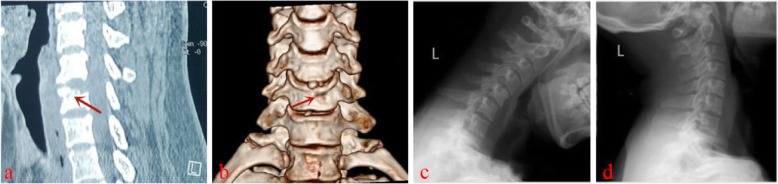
A 6-month postoperative CT examination revealed that the bone tunnel had healed and no fracture occurs (a, b). The last follow-up X-ray radiographs in flexion and extension positions of cervical spine(c, d) showed that the physiological curvature of the cervical spine remained good and there was no cervical spine instability
